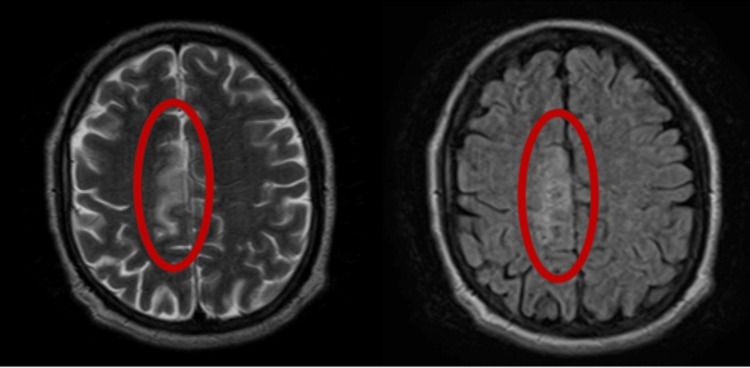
Brain MRI (right and left red circles) showed moderate-sized infarct in the right medial frontal parietal pericallosal region, concerning for right anterior cerebral artery territory infarct; no hemorrhage was observed
